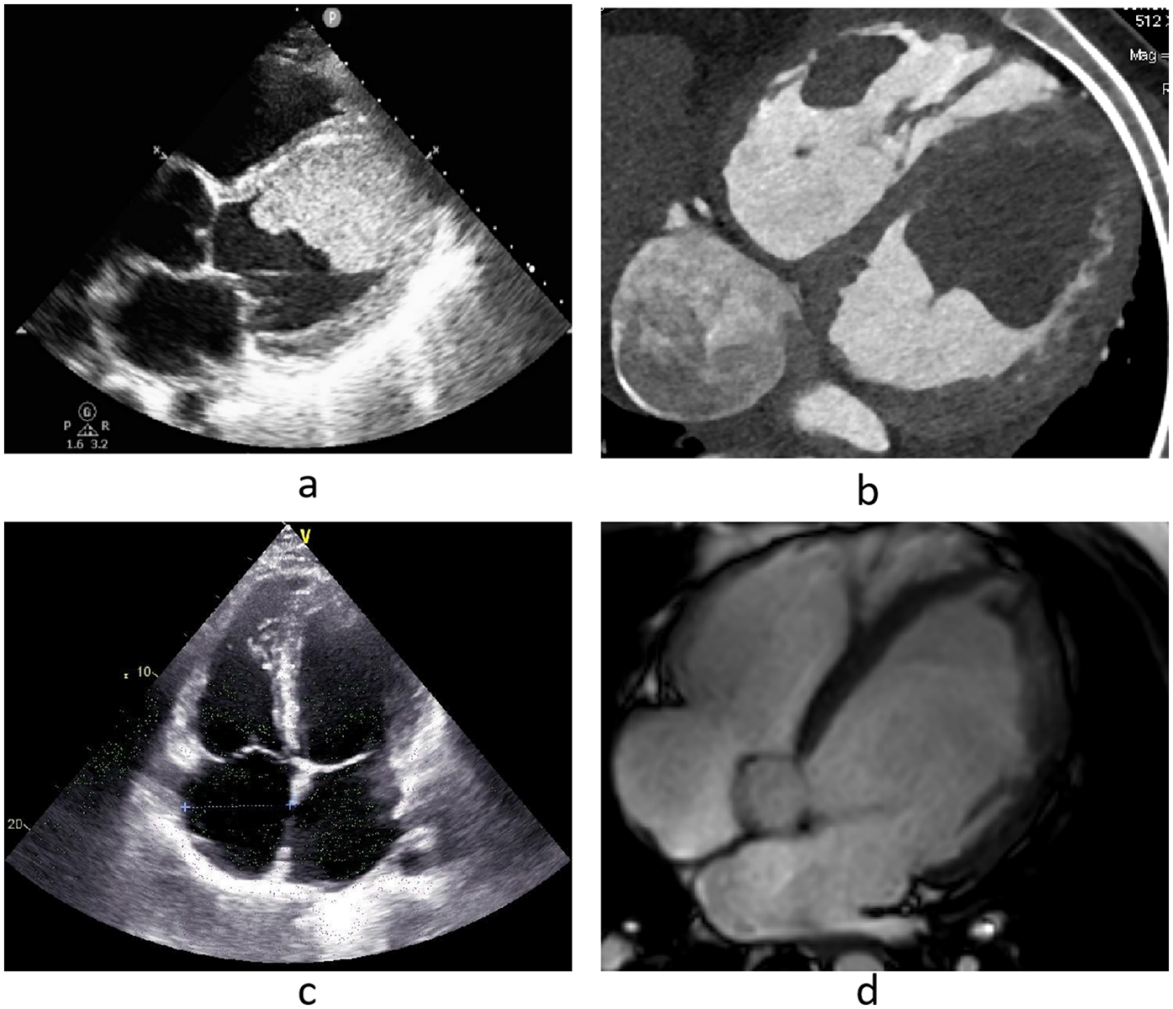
(a,b) Echocardiography and cardiac CTA on admission, (c,d) echocardiography and cardiac MRI before discharge.
